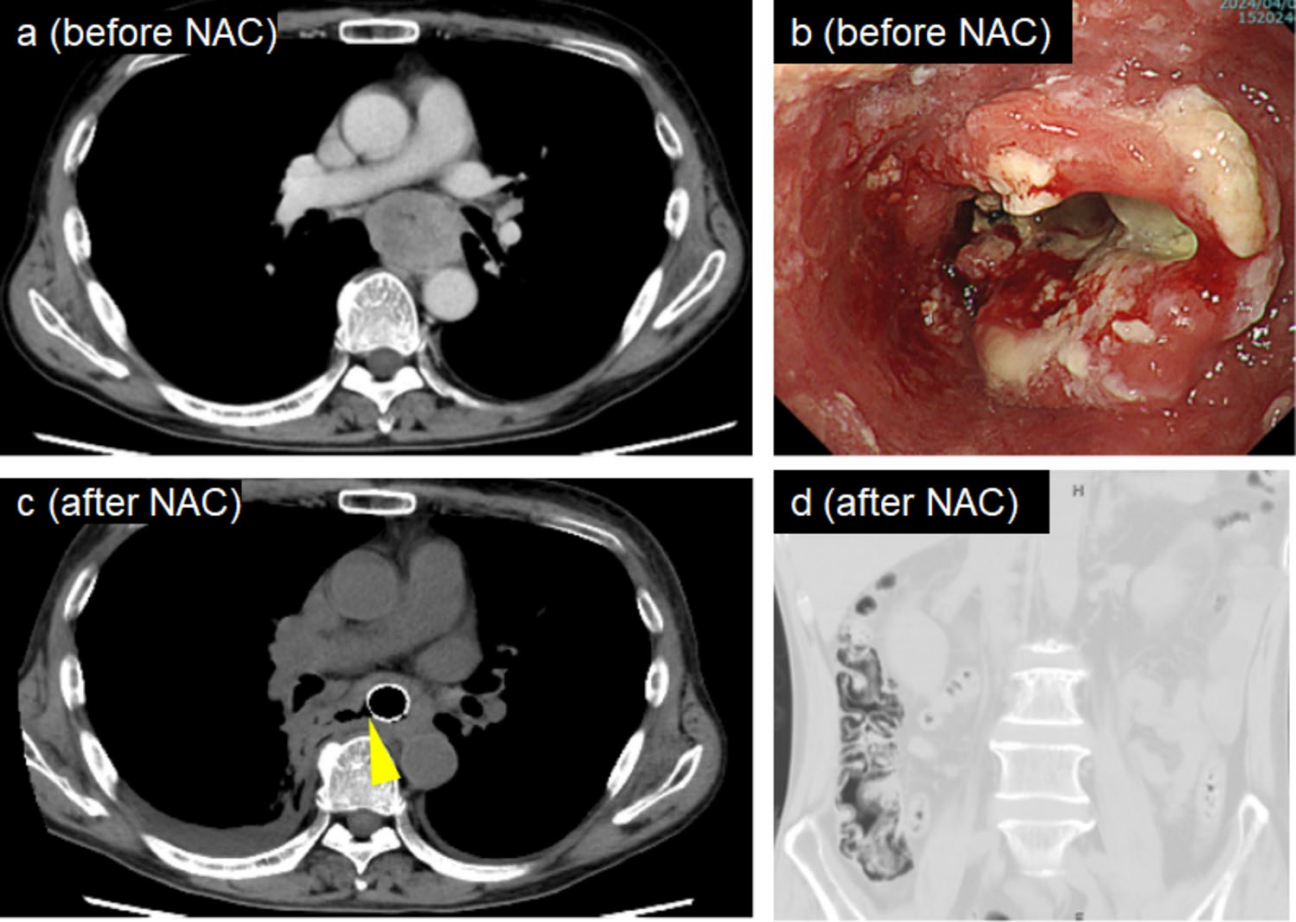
Clinical course images of Case 2. (a) Contrast-enhanced CT scan showing a tumor in the middle thoracic esophagus. (b) Endoscopic image showing a type 3 tumor in the middle thoracic esophagus. (c) CT scan showing right-sided pyothorax and an esophagorespiratory fistula (arrowhead). (d) CT scan showing pneumatosis intestinalis at ascending colon. NAC: neoadjuvant chemotherapy
